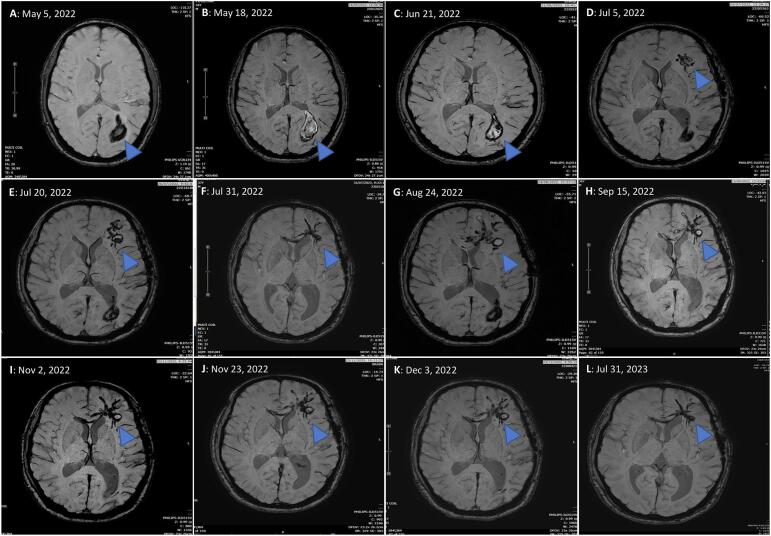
(A-L) MRI images of the patient's brain in series: Fig. A showed intraparenchymal hemorrhage in the left parietooccipital lobe (arrowhead) with mild perilesional edema; Figs. B, C showed a reduction in the size of occipital lobe hematoma two weeks pre-craniotomy (arrowheads), along with a new rim-enhancement lesion with a more invasive serpiginous hemorrhagic tract in the left temporal lobe; Fig. D showed brain edema in the left temporal lobe (arrowhead) following the definite diagnosis, the treatment with albendazole along with intravenous dexamethasone was administered, then treatment was stopped and ivermectin was administered alternatively, Figs. E showed progression of serpiginous hemorrhagic lesions, Figs. F-J showed stable serpiginous hemorrhagic lesions during combined treatment using albendazole and ivermectin for full four courses subsequently, Fig. K showed serpiginous hemorrhagic tract ceased, indicating the cessation of *Gnathostoma* larval worm migration, Fig. L showed no new serpiginous hemorrhagic tracts and improved necrotic lesions in the patient's brain.
